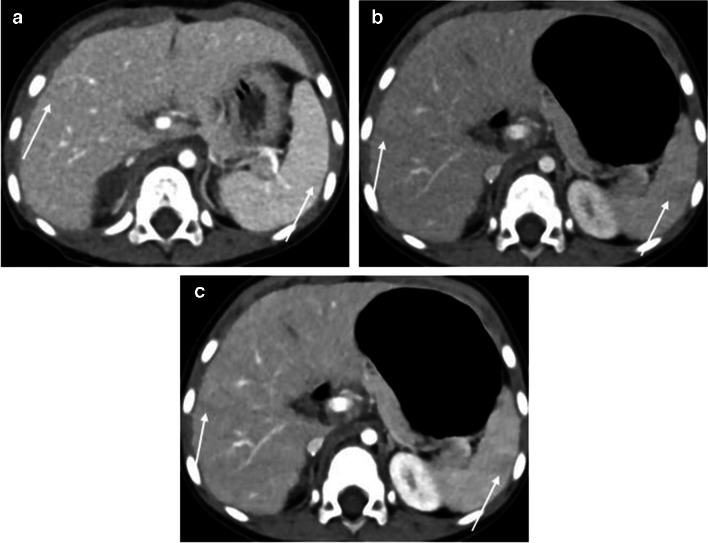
Axial contrast-enhanced computed tomography (CT) images of a 2-year-old girl with immature teratoma. Sequential abdominal CT was conducted within 4 months. a Standard-dose image shows a minimal streak artifact underneath both ribs (*arrows*). b Low-dose image shows a mild streak artifact underneath both ribs (*arrows*). c In the contrast-boosted image, the streak artifact is more prominent in the more enhanced liver and spleen (*arrows*). The qualitative artifact scores of the standard-dose, low-dose, and contrast-boosted images were 5/4, 4/4, and 3/3, respectively (reader 1’s score/reader 2’s score)
